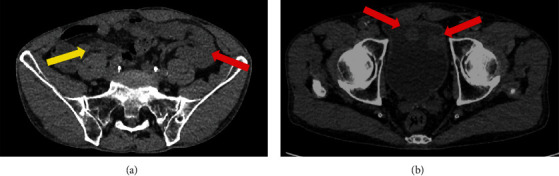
CT scan with contrast 2017. (a) Prior kidney transplant (red) and pancreas transplant (yellow). (b) Multiple masses in the bladder.
